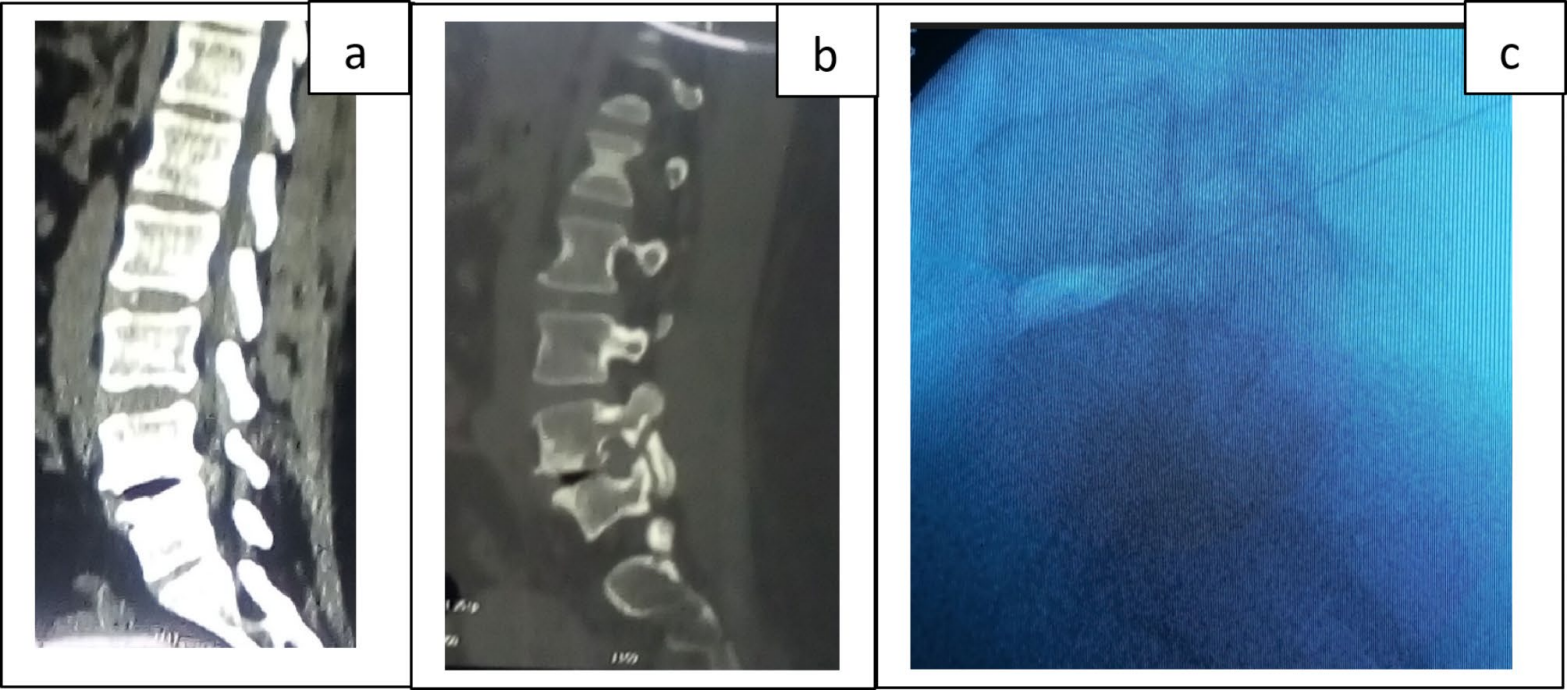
CT and per discolysis fluoroscopy images of the patient of the observation 1. CT sagittal reconstruction, parenchymal window, passing through the spinal canal (a) showing a disc vacuum L5S1 and a herniated L5S1 on an S1 lumbalization; CT in sagittal reconstruction, bony window passing through the posterior articular (b) visualizing an L5S1 disc vacuum and an undisplaced isthmic lysis of L5; image of fluoroscopy during the discolysis procedure (c) objectifying the needle of the discolysis implanted in L5S1 and the opacity of the ozone that was injected
